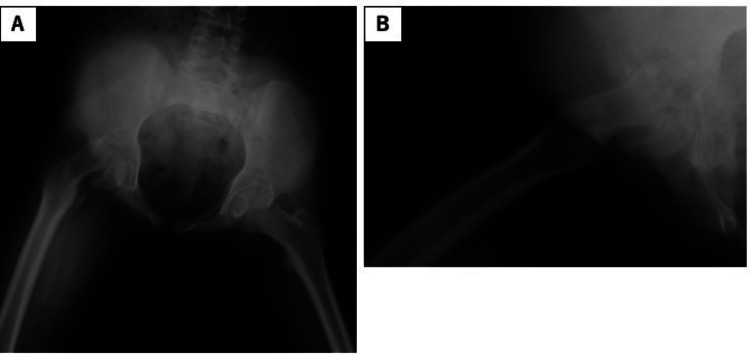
Radiograph (taken at our hospital) revealing more severe slippage, manifesting as a head-shaft angle of 91° and the posterior tilting angle of 87° (arrow). (A) Anteroposterior view; (B) lateral view.
